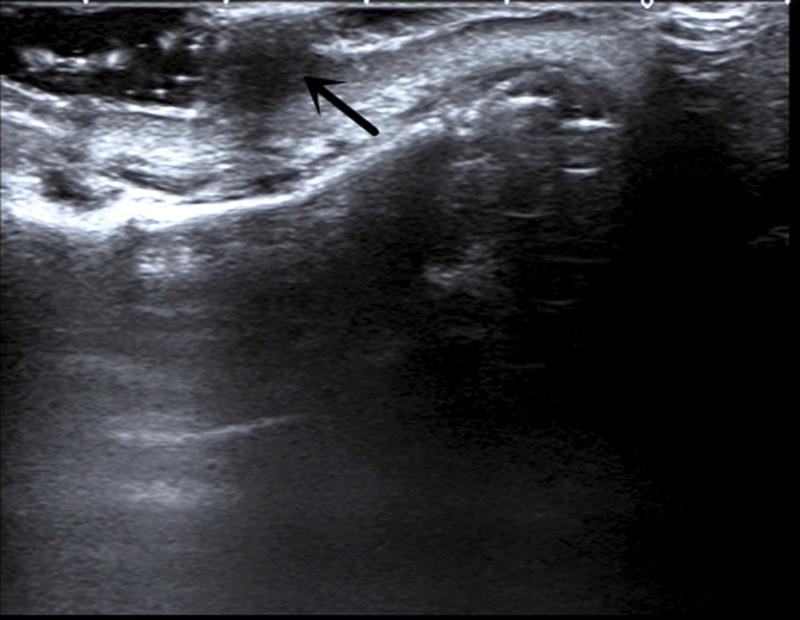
Two-dimensional US shows hypoechoic mass with uniform echo within the skin layer with clear boundaries (black arrow). US = ultrasound.
